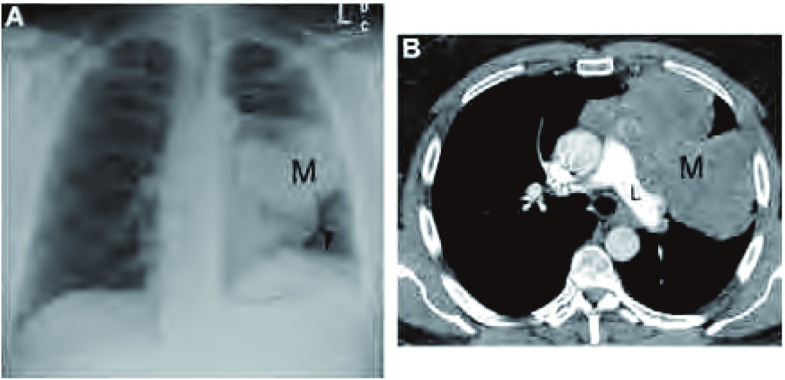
侵犯膈神经。A：后前位胸片显示，巨大的纵隔胸腺瘤（M），伴有左侧的膈肌抬高（箭头所示）。B：胸部增强CT（软组织窗）显示，左肺动脉（L）水平胸腺瘤（M）占据整个左侧肺门，该部位是左侧膈神经的走行区域。 Phrenic nerve involvement. A: Posterior anterior chest radiograph of newly diagnosed thymoma demonstrates a large mediastinal mass (M) with associated elevation of the left hemidiaphragm (arrowhead). B: Contrast-enhanced chest computed tomography (CT) (soft tissue window) at the level of the left pulmonary artery (L) demonstrates the thymoma (M) abutting the entire left side of the mediastinum anterior to the hilum, this location is in the expected course of the left phrenic nerve.
